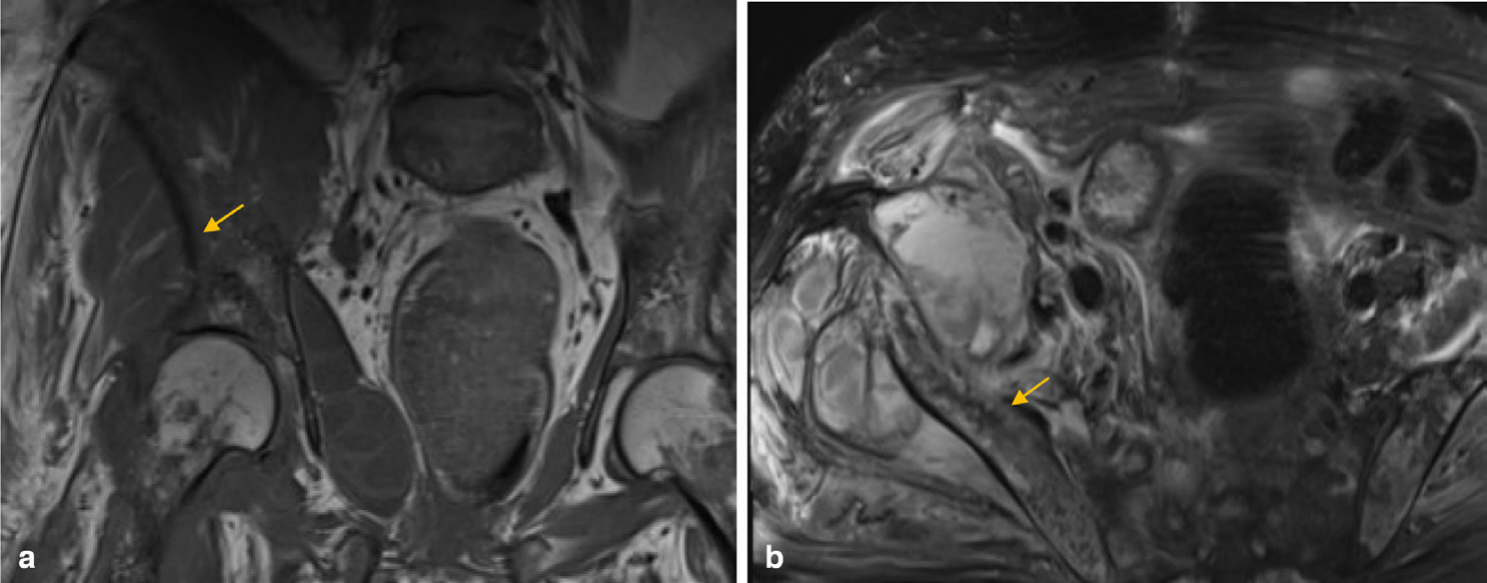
Previous coronal T1 (a) and axial fat saturated T2 (b) weighted images of the pelvis indicate multiple small T1 and T2 hypointense foci (arrows), corresponding to the intraosseous gas seen on CT scan.
